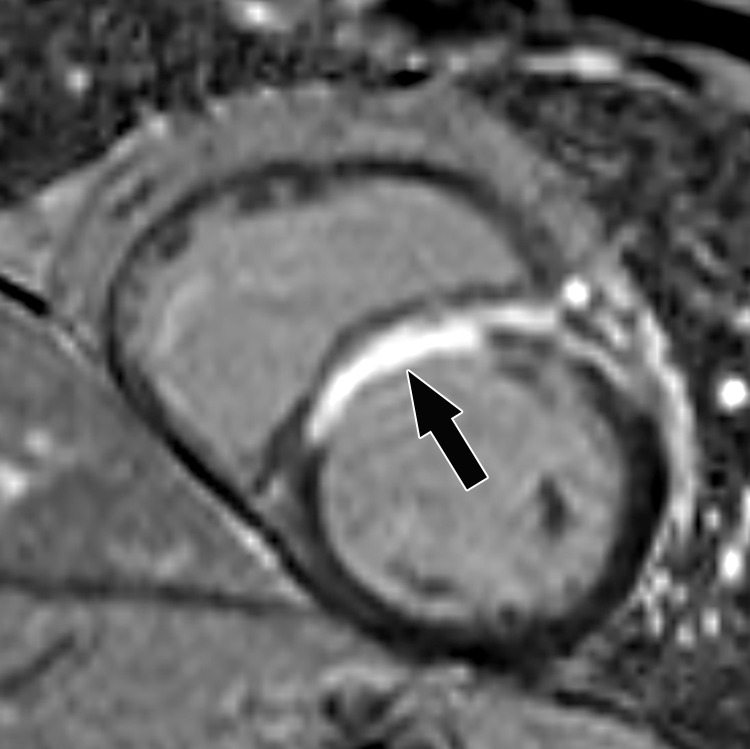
Subendocardial enhancement in a 44-year-old man with a history of CAD, MI, and tobacco use who was referred for cardiac MRI to assess MI size. Short-axis LGE cardiac MR image of the LV shows subendocardial-based enhancement, with about 75% transmural extent within the basal septal wall (arrow), as well as some extension into the adjacent anterior wall. The transmural extent of enhancement is greater than 50%, which indicates a lower likelihood of recovery with revascularization.
